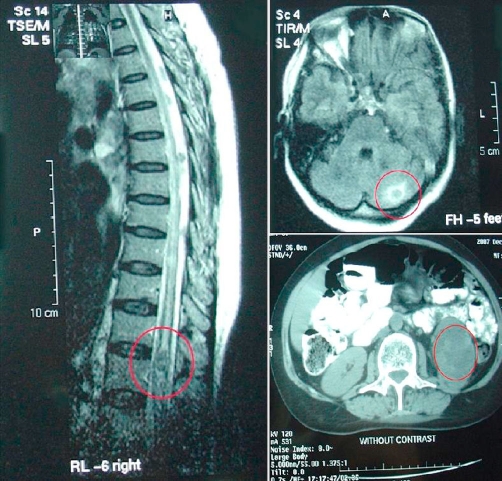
MR imaging of the spine at the level of conus medullaris. A non enhancing cystic lesion is seen in the area.
